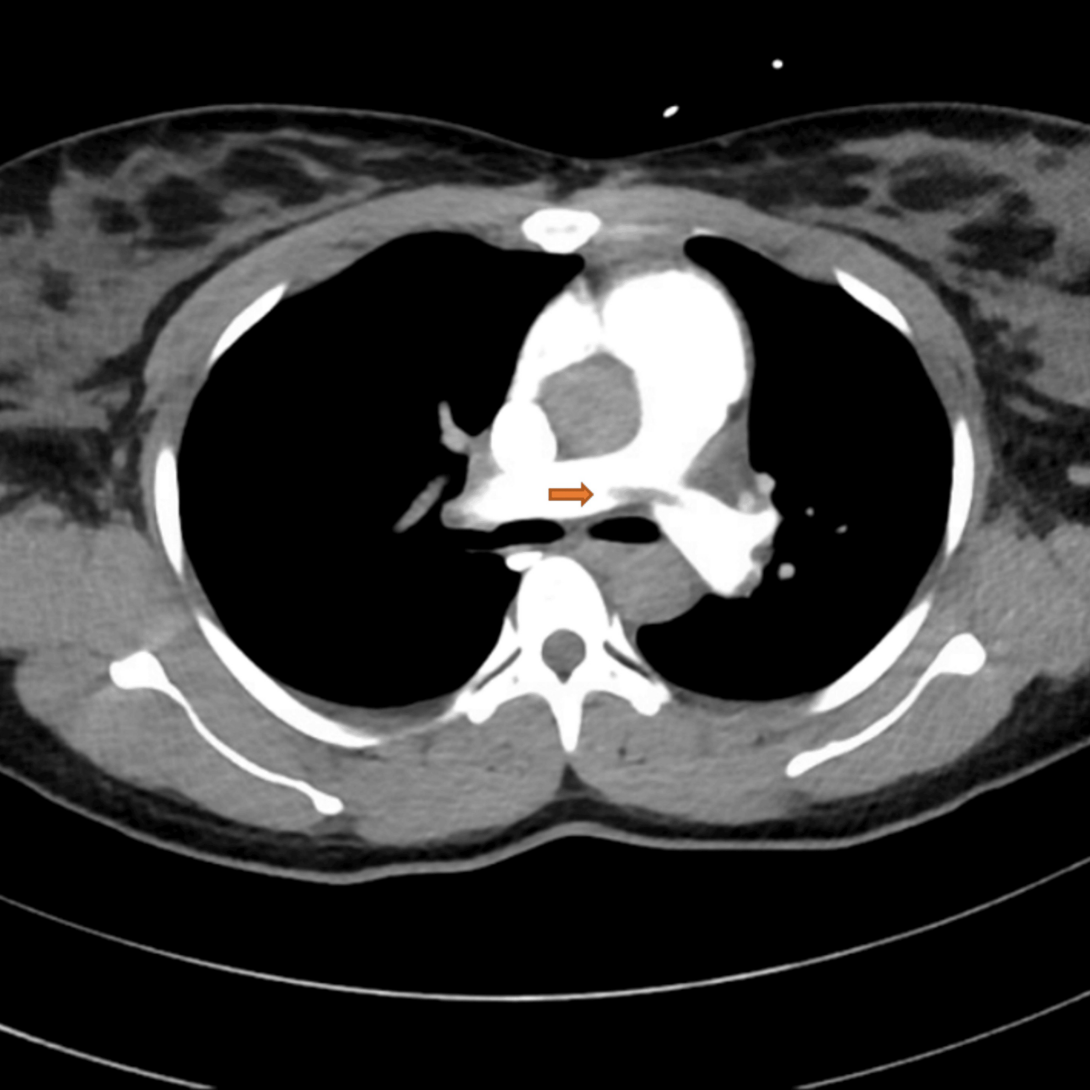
Computed tomography pulmonary angiography (CTPA) showing saddle embolus at the pulmonary trunk bifurcation.
